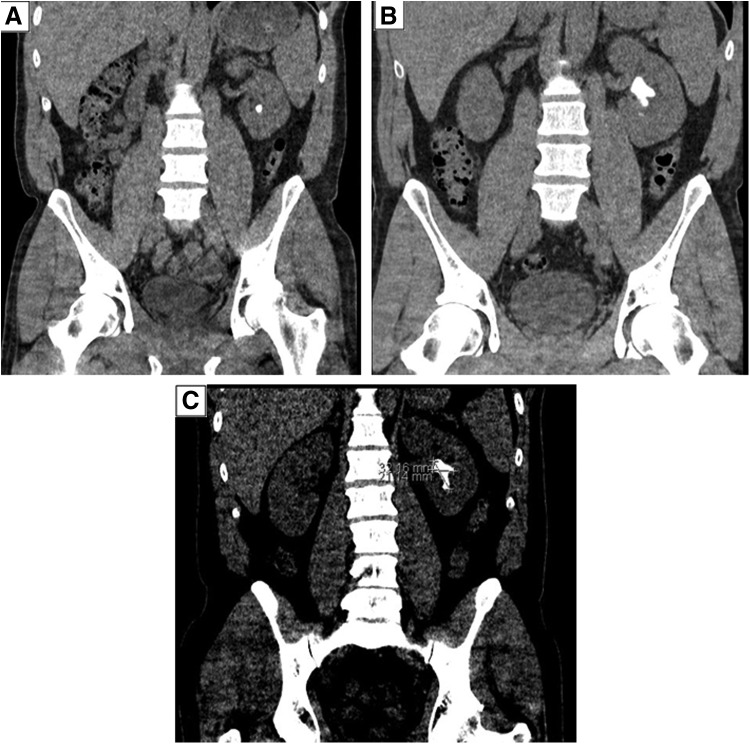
CT scan showing the progression of the left renal calculus from two 5 mm stones to a large staghorn calculus. (A) Noncontrast CT scan showing the small left lower pole renal calculus. (B) Noncontrast CT scan showing the 3.4 × 2 cm left staghorn calculus. (C) Noncontrast CT scan showing the remaining staghorn calculus after failed left ureteroscopy laser lithotripsy measuring 3.2 × 2.1 cm.
